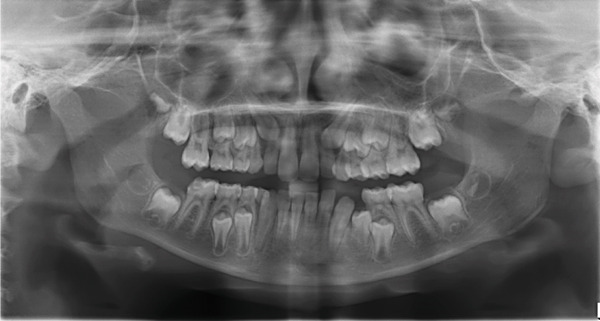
Pretreatment radiographic assessment panoramic radiograph.
